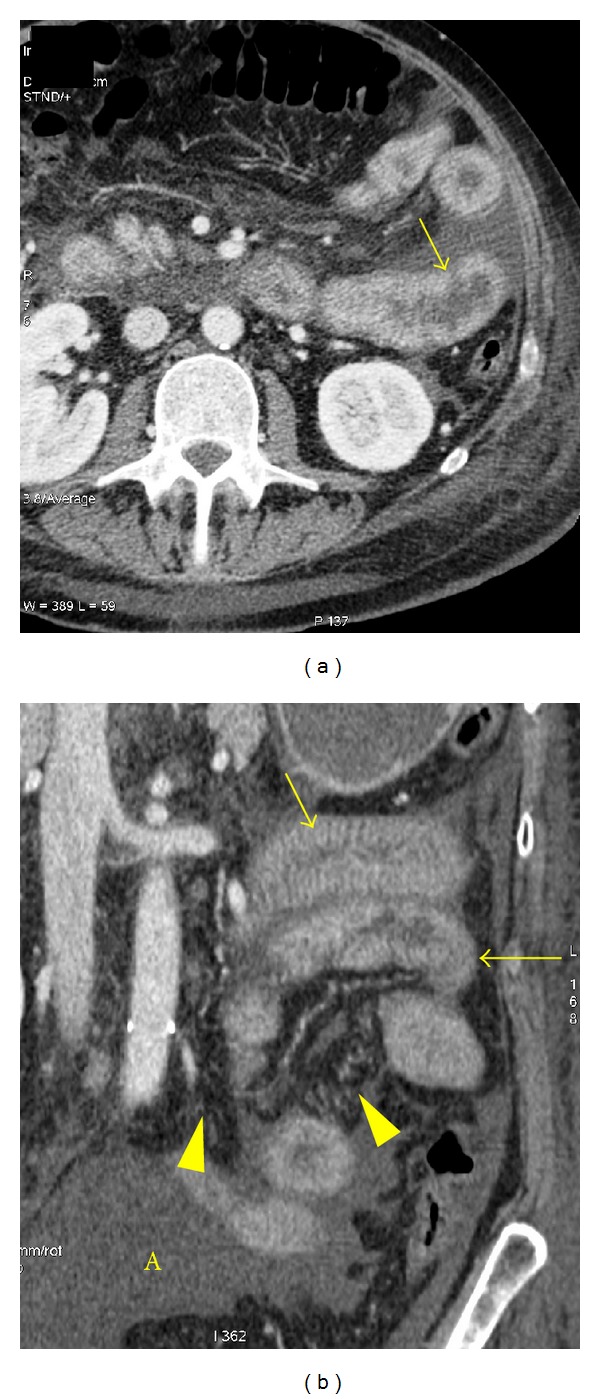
Small bowel wall thickening in a patient with hypoproteinemia due to hepatic cirrhosis. Axial (a) and coronal (b) contrast-enhanced CT images show diffuse thickening of the bowel wall and folds (arrows). Ascites (A) and mesenteric edema (arrowheads) are noted.
